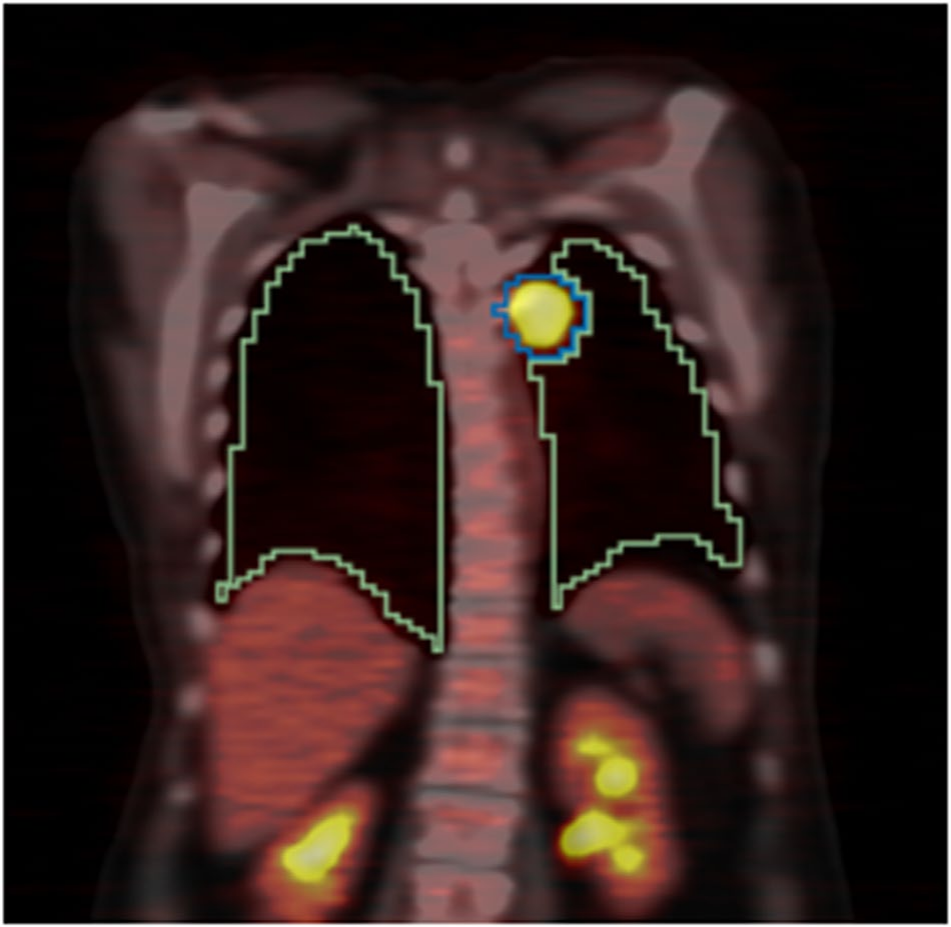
Automatic segmentation of Lung (green) and FDG-avid tumor (blue) from FDG-PET/CT scans of patient RIDER-2610856938.
